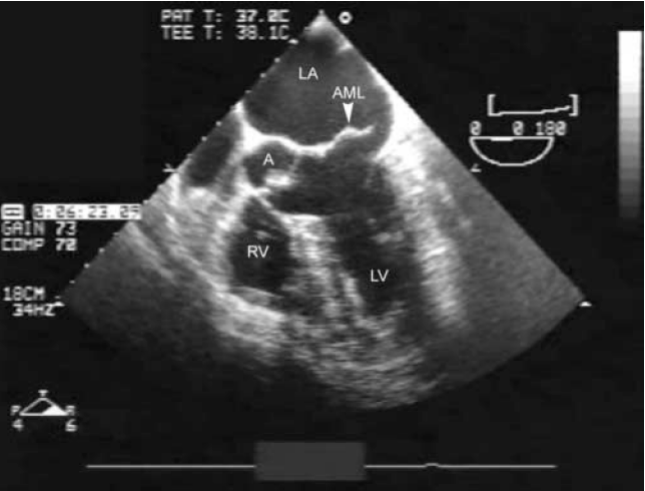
Transesophageal Echocardiogram shows ruptured chordae (C) of anterior mitral leaflet (AML) prolapsing into LA.
